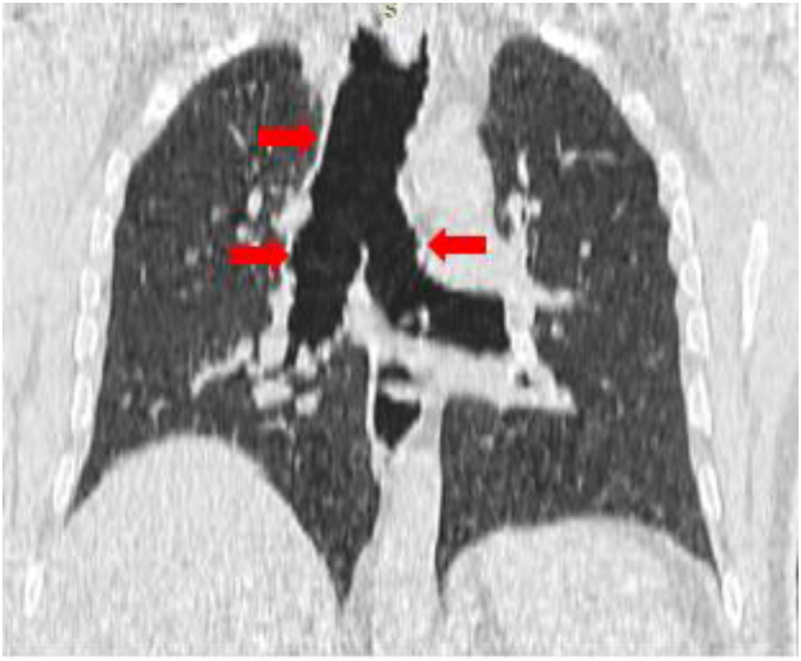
Chest CT scan: coronal reconstruction in a plane passing through the trachea and main bronchi showing tracheobronchomegaly.
